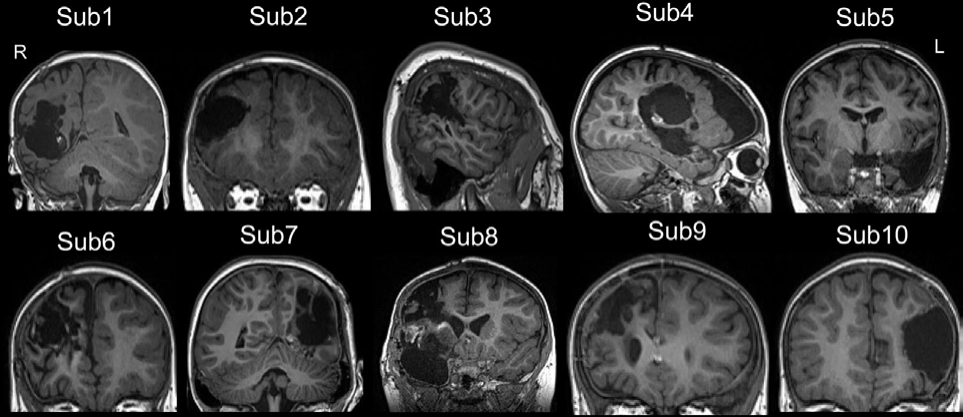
The post-surgical structural image of all patients. All images were achieved from the high-resolution T1-weighted 3D images. The images were showed in coronal or sagittal view of each subject. Surgery location: right hemisphere (Sub 1–4, Sub 6, Sub 8, and Sub 9), left hemisphere (Sub 5, Sub 7, and Sub 10). The detail of epilepsy type and the clinical information can be seen in Table 1. L, left; R, right.
